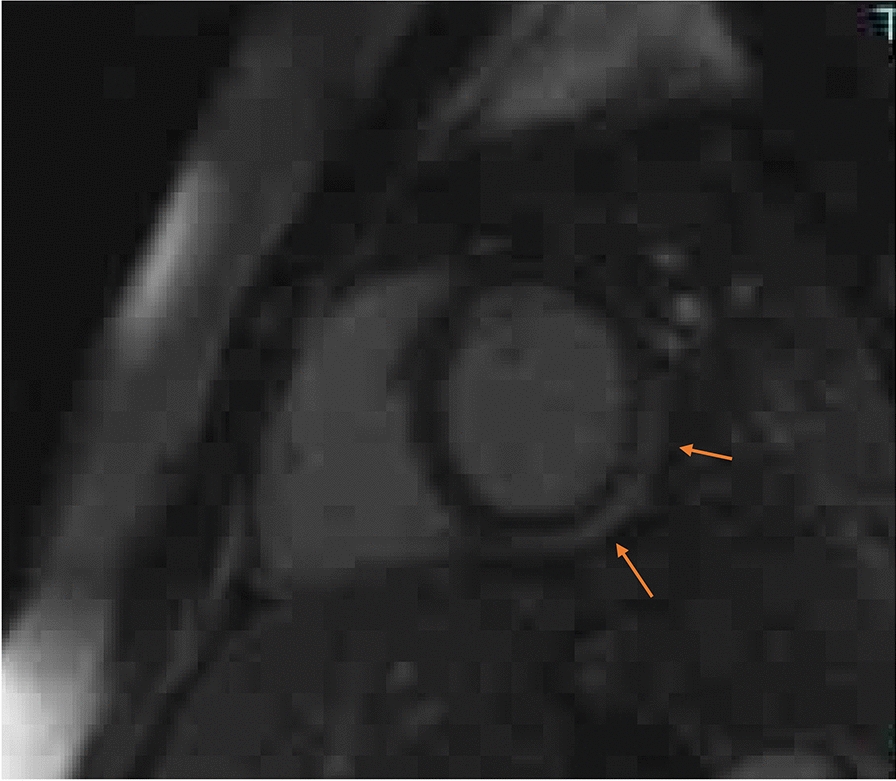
Cardiac magnetic resonance imaging (CMR) showed epicardial enhancement of the posterolateral LV wall as a sign of a myocarditis
